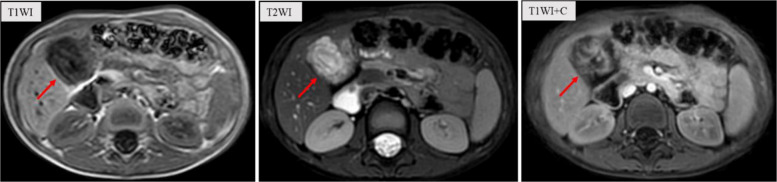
Abdominal MRI demonstrated an enlarged gallbladder
